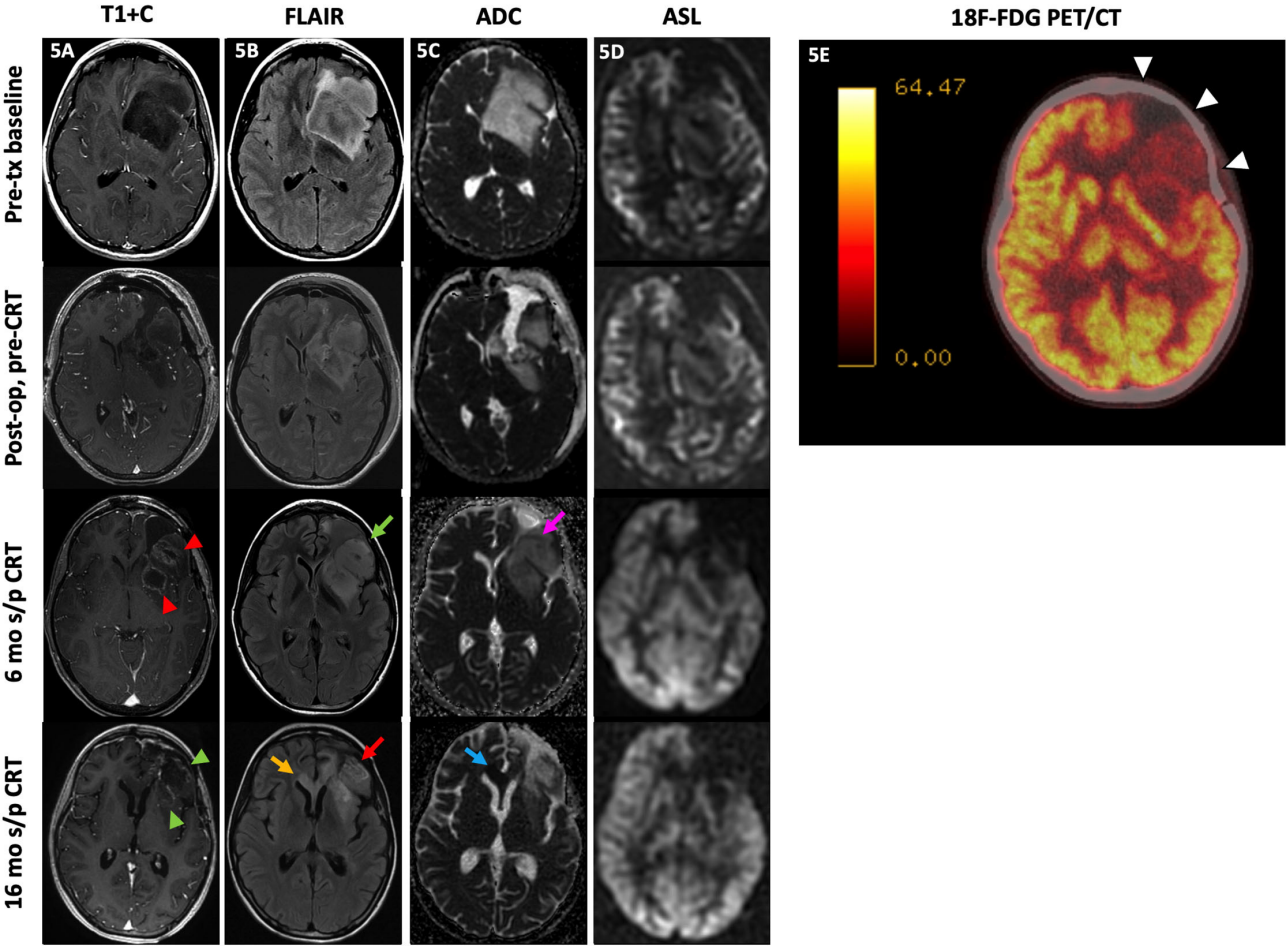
Treatment effect. A 17-year-old female with anaplastic astrocytoma (IDH wt, MGMT+) initially presented with a predominantly non-enhancing left frontal lobe mass [(A), top row] with extensive locoregional mass effect, mild perilesional FLAIR hyperintensity [(B), top row], and no substantial diffusion restriction [(C), top row] or ASL hyperperfusion [(D), top row]. After subtotal resection, there was no substantial enhancement [(A), 2nd row] with mild residual FLAIR hyperintensity [(B), 3rd row] without diffusion restriction [(C), 3rd row] or ASL hyperperfusion [(D), 4th row]. At 6 mo s/p CRT, new nodular enhancement was seen at the resection bed margins [(A), 3rd row, red arrowheads] with increased expansile FLAIR hyperintensity [(B), 3rd row, green arrow], mild diffusion restriction at the anterolateral aspect of the resection margin [(C), 3rd row, pink arrow], and persistent lack of hyperperfusion on ASL images [(D), 3rd row]. Subsequently, the patient underwent a ^18^F-FDG PET/CT a month later which showed hypometabolism throughout the left frontal resection cavity favored to represent treatment effect [(E), white arrowheads]. Much later at 16 mo s/p CRT, the left frontal resection cavity showed decreased enhancement [(A), 4th row, green arrowheads] with decreased FLAIR signal [(B), 4th row, red arrow] at the areas of prior signal abnormality; however, there is a new focal area of FLAIR hyperintensity at the corpus callosum crossing midline [(B), 4th row, yellow arrow] with diffusion restriction [(C), 4th row, blue arrow] and no ASL hyperperfusion [(D), 4th row]. These findings were consistent with delayed Psp/treatment effect at the left frontal resection cavity, which was supported by the PET/CT result, with a new area of probable recurrence at the corpus callosum. The patient did not undergo biopsy and passed away before additional follow-up imaging was obtained. ADC, apparent diffusion coefficient; ASL, arterial spin labeling; CRT, chemoradiotherapy; FDG, fluorodeoxyglucose; FLAIR, fluid-attenuated inversion recovery; mo, months; op, operative; PET/CT, positron emission tomography/computed tomography; s/p, status post;T1+C, post-contrast T1-weighted images; tx, treatment.
